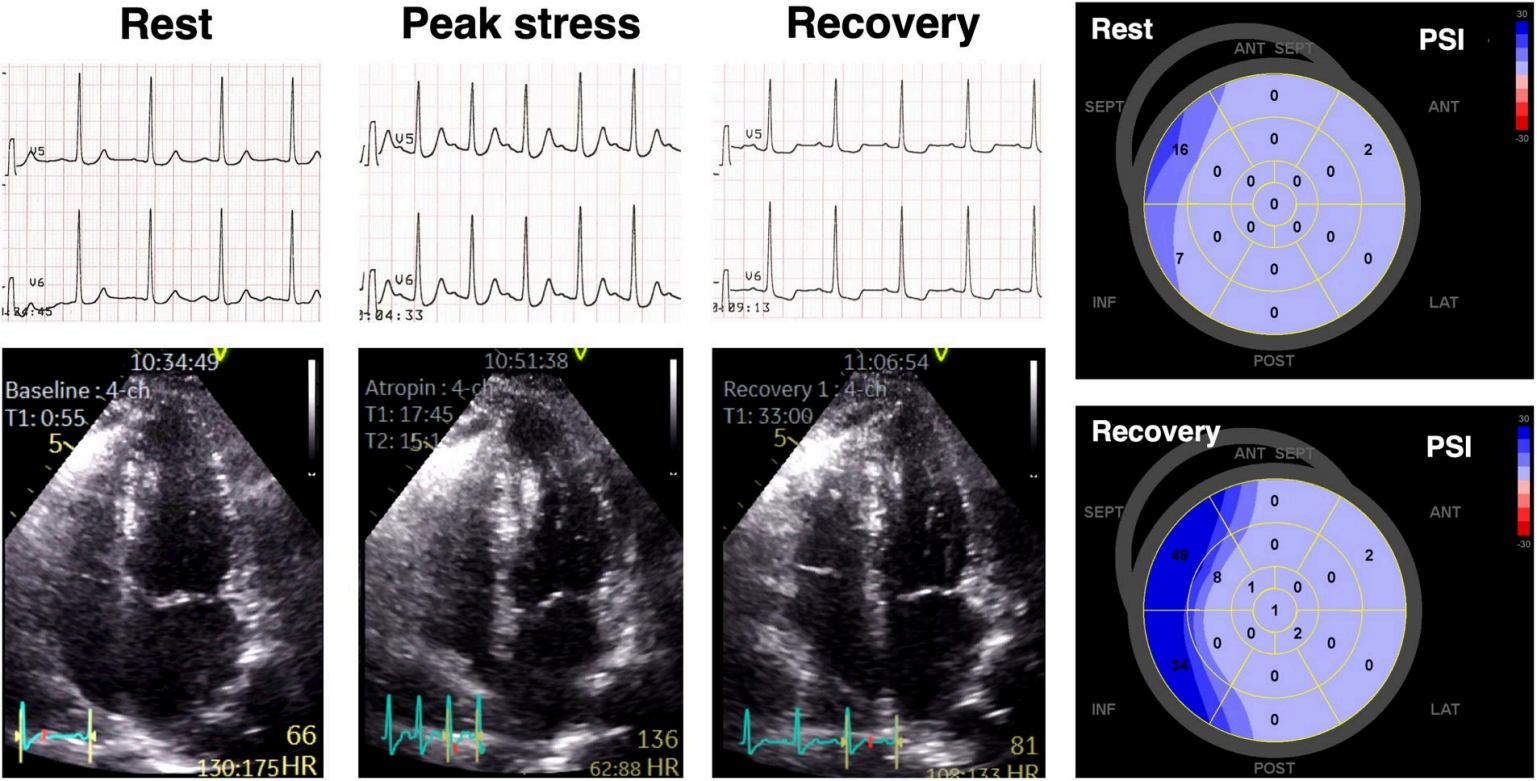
An example of a patient presenting with anginal symptoms and significant ST-segment depressions during dobutamine stress echocardiography (DSE), but with no obstructive coronary artery disease detected on invasive coronary angiography. Although there were no wall motion abnormalities observed during DSE, significant abnormalities in post-systolic myocardial deformation were evident, as indicated by elevated PSI values in multiple left ventricular segments.
